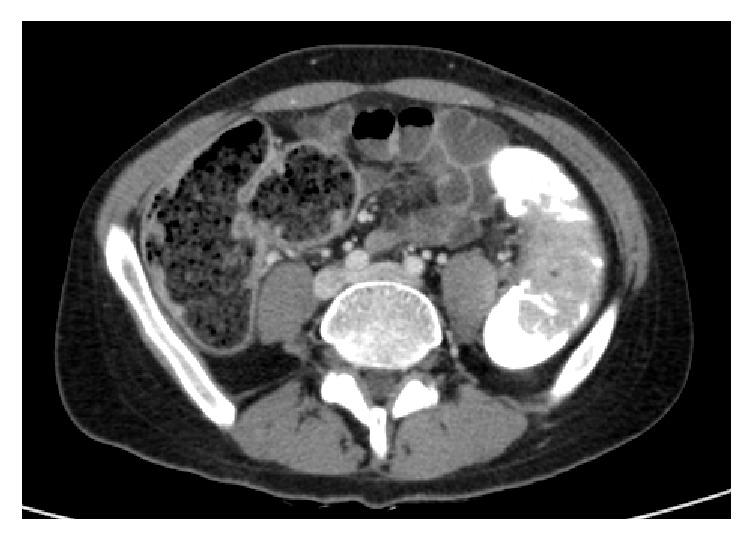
Abdominal CT scan showing GIP the left colon.
